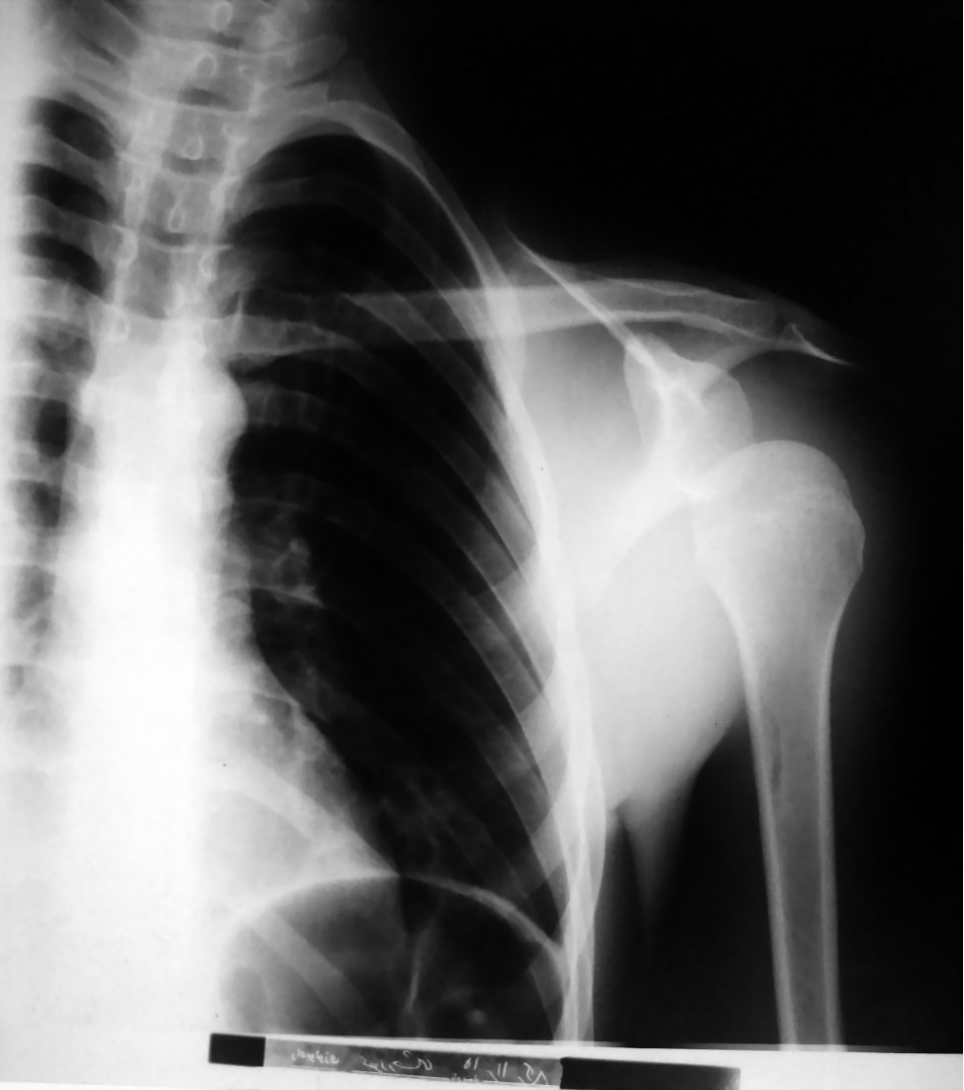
AP x-ray of the left shoulder shows anteroinferior dislocation.
